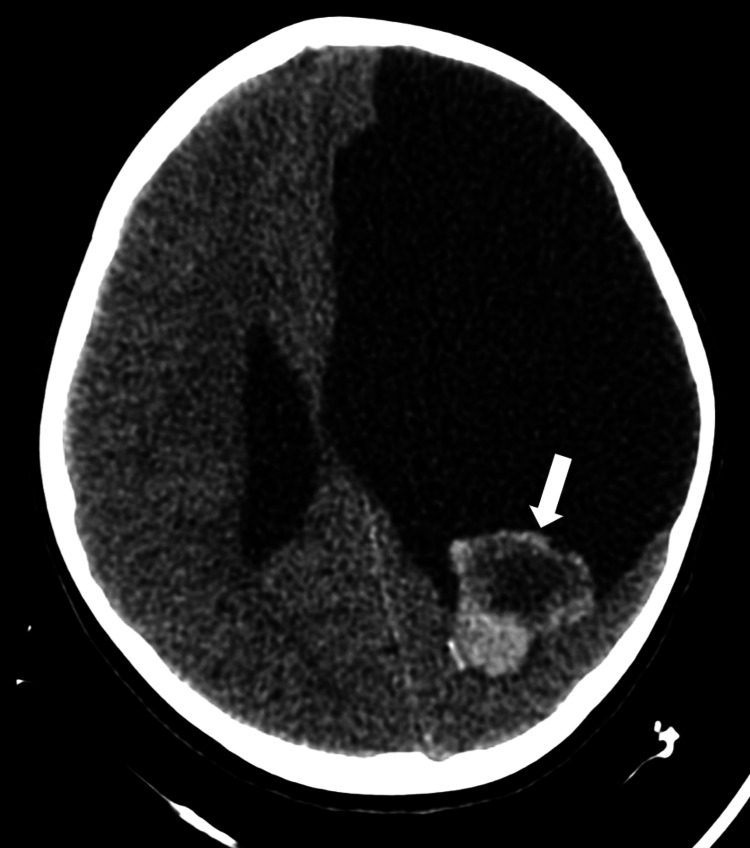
Initial axial non-contrast head CT. A heterogeneous hyperdense lesion measuring 3.1 x 2.9 x 3.3 cm was identified (arrow).
